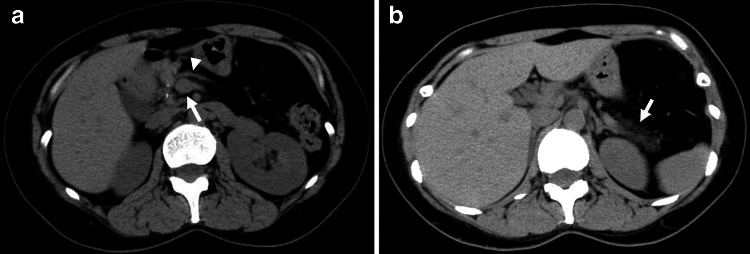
Images from 3-mm slice abdominal CT scans obtained in December 2013, 9 years after starting OLT (at 42 years of age). a
